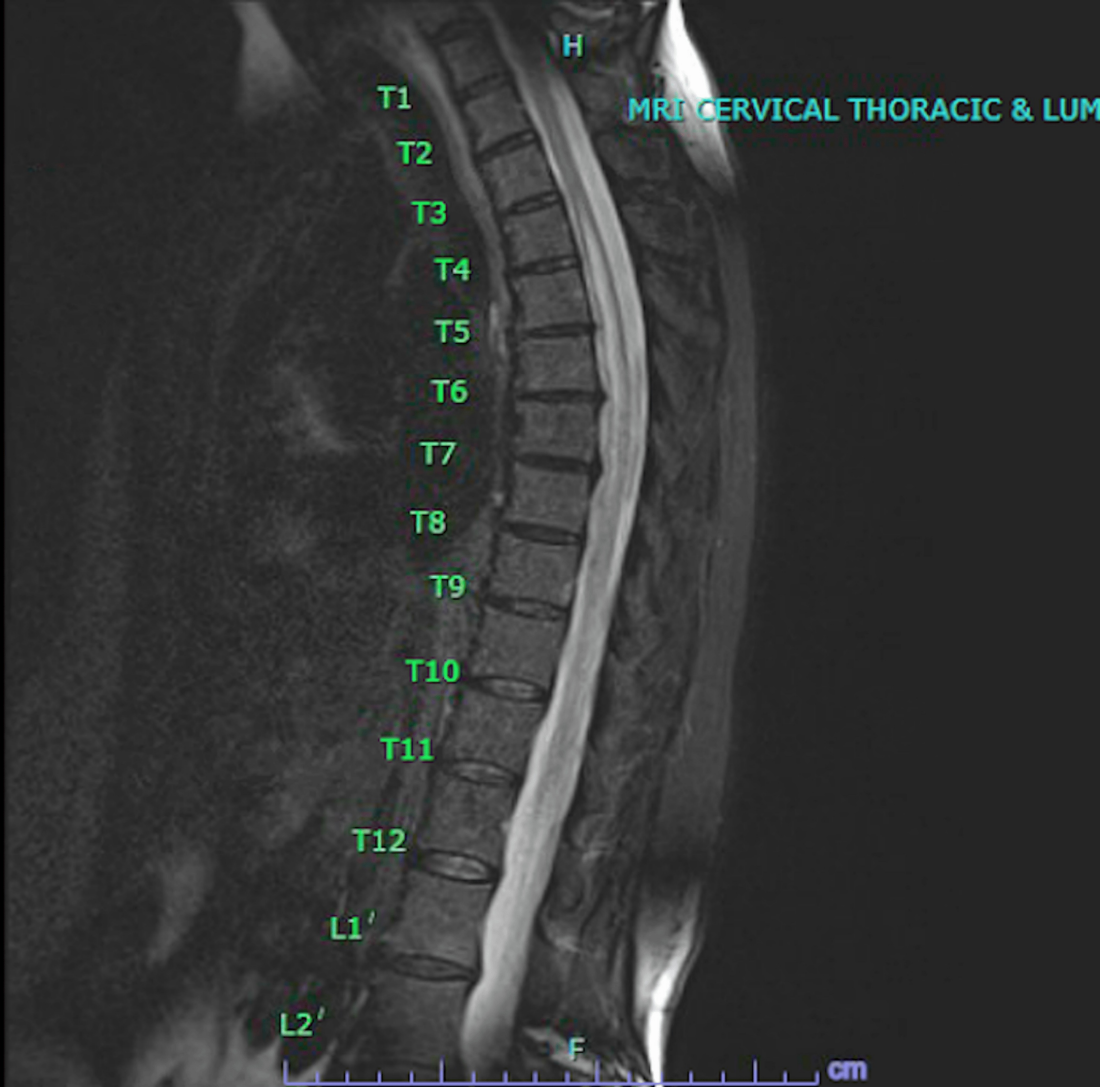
MRI cervical/thoracic/lumbar spine without contrast showed possible syrinx from T3-T12
